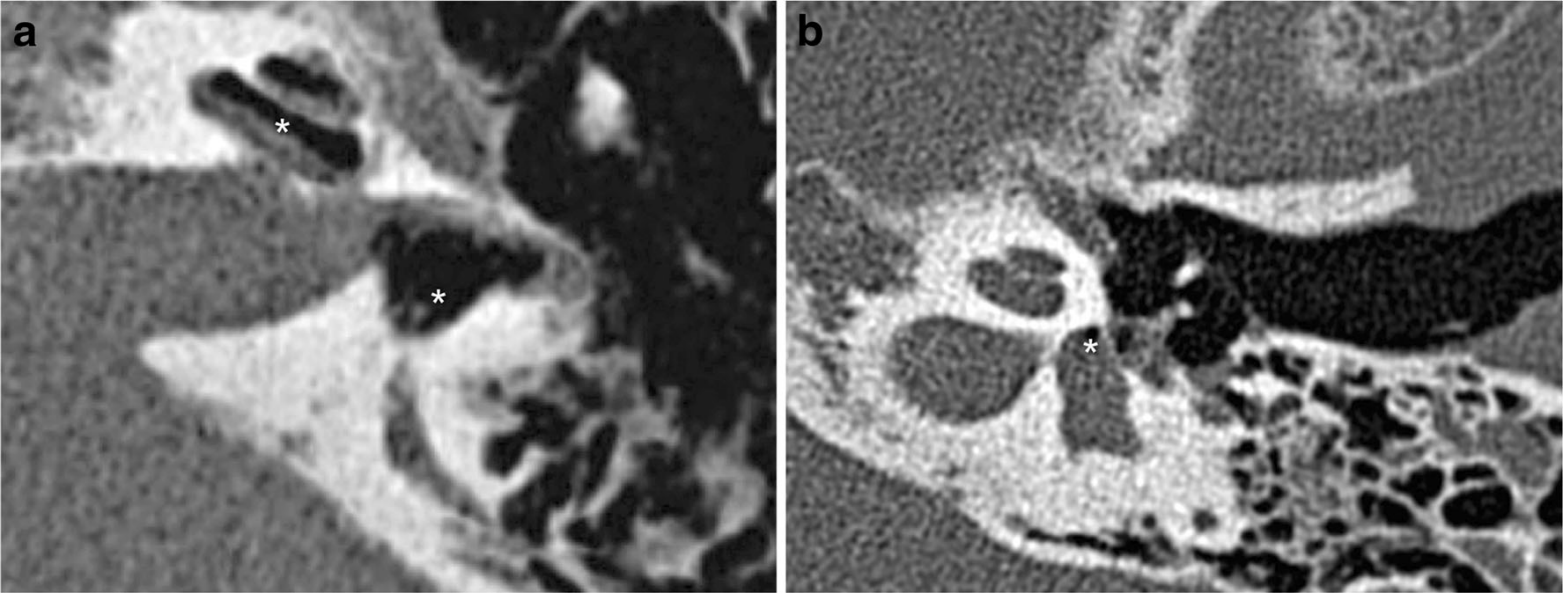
a Perilymphatic fistulae: CT axial views showing a perilymphatic fistula, highlighted by a pneumolabyrinth (*white stars*). Perilymphatic liquid has leaked into the middle ear. Massive pneumolabyrinth was seen 1 month after a translabyrinthine fracture, with air in the perilymphatic space (scala vestibuli and the vestibule) b Example of pneumolabyrinth without temporal bone fracture (*white star*)
